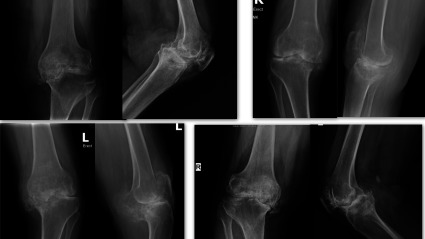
Pre-operative X-rays of patients with severe varus deformity and extensive bone loss from posteromedial condyle.
